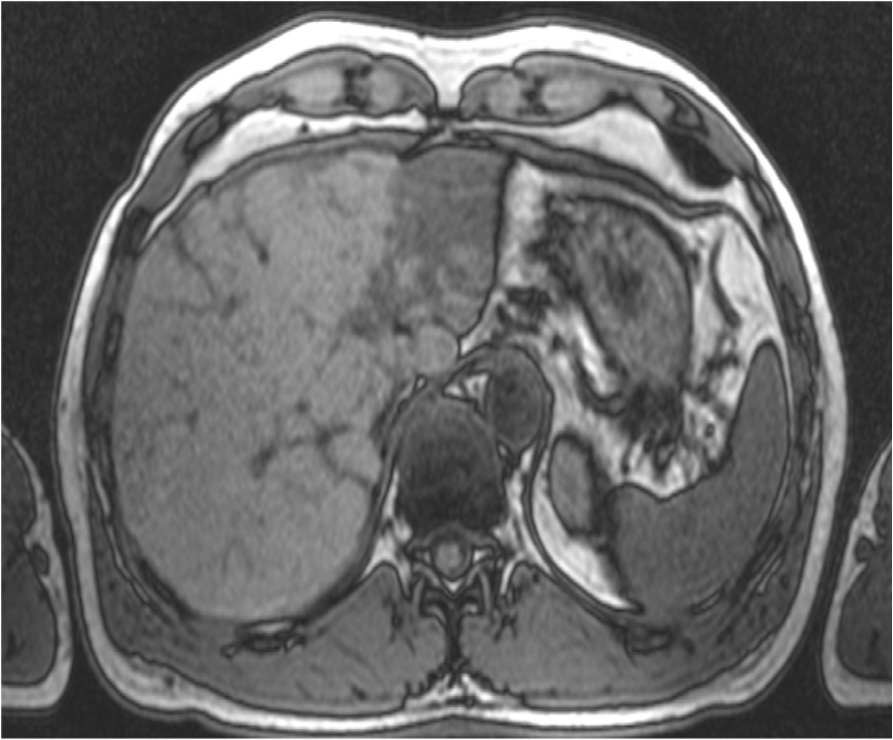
MRI of the liver. One month after discontinuing adjuvant treatment MRI shows clearly cirrhotic changes of the parenchyma.
